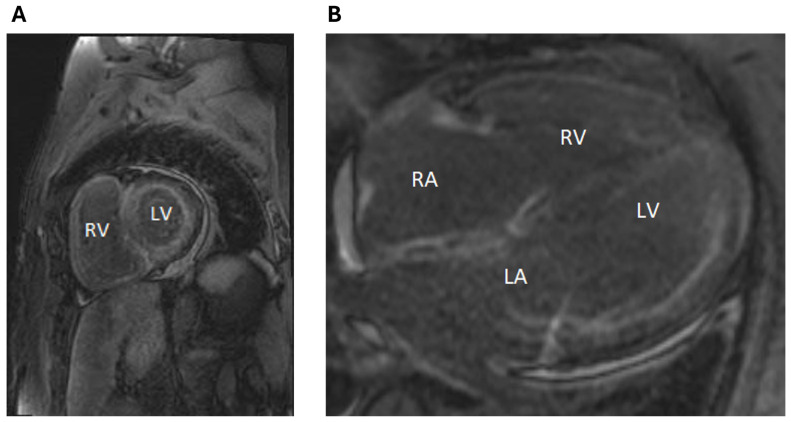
Cardiac Magnetic Resonance Imaging (MRI) findings. Panels (A,B) are delayed-phase images on cardiac MRI in the short-axis, and 4-chamber views, respectively, demonstrating diffuse late gadolinium uptake throughout the left and right ventricular myocardium as well as in the atrial free walls, consistent with infiltrative process such as cardiac amyloid. Abbreviations: LA—left atrium, LV—left ventricle, RA—right atrium, RV—right ventricle.
